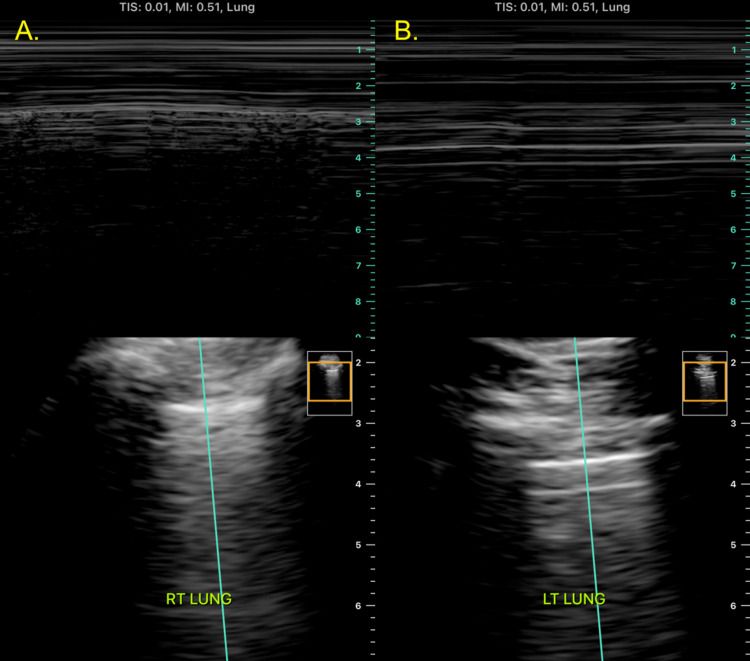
Lung ultrasound: M-mode A. Ultrasound of the right lung apex with M-mode demonstrating a "seashore sign" seen in the presence of lung sliding. B. Ultrasound of the left lung apex with M-mode demonstrating a "barcode/stratosphere sign" seen in the absence of lung sliding. This is suggestive of a pneumothorax.
